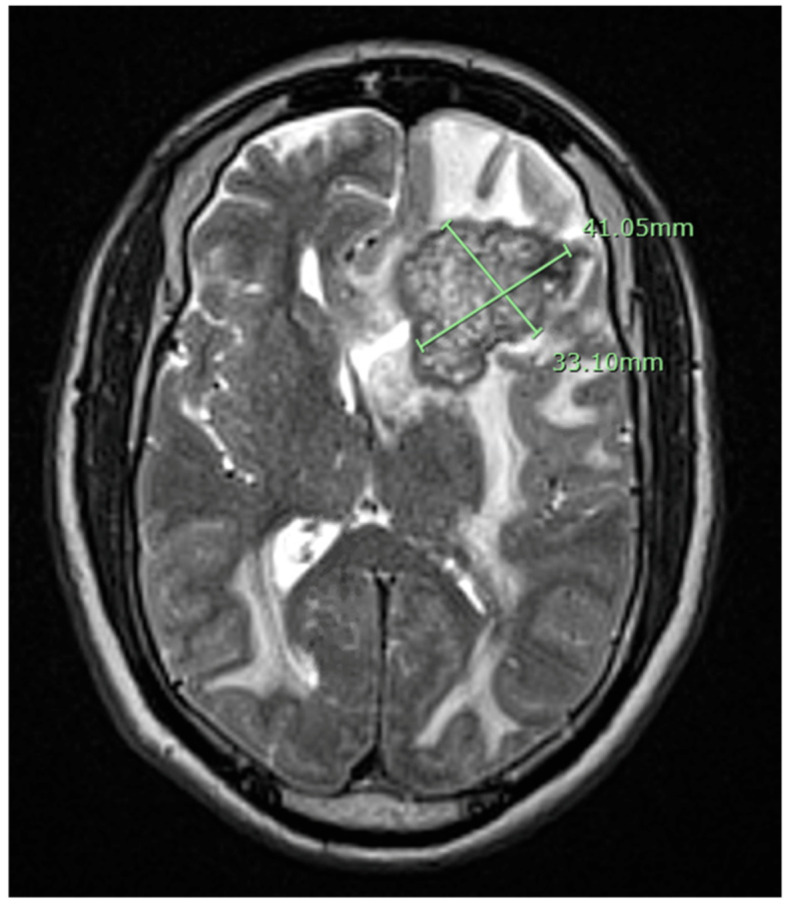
The magnetic resonance imaging (MRI) of the brain with gadolinium contrast showed a 41 mm × 33 mm heterogeneous peripherally enhancing lesion in the left frontal lobe with vasogenic edema. It shows mass effect including 13 mm of left to right midline shift and compression of the left frontal horn.
